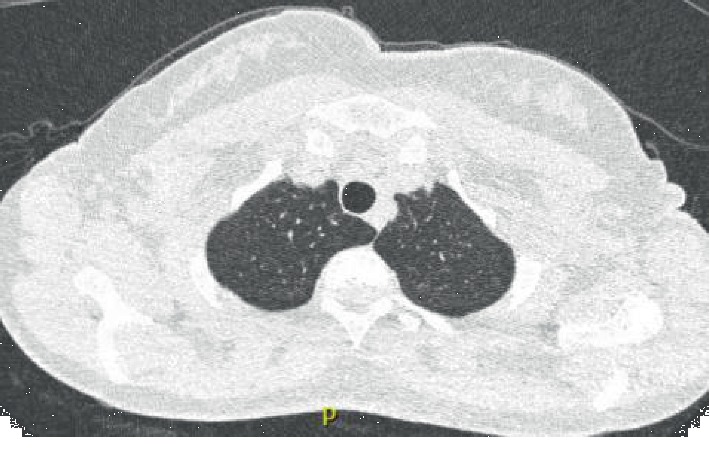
HRCT chest, prone image, and apical view.
